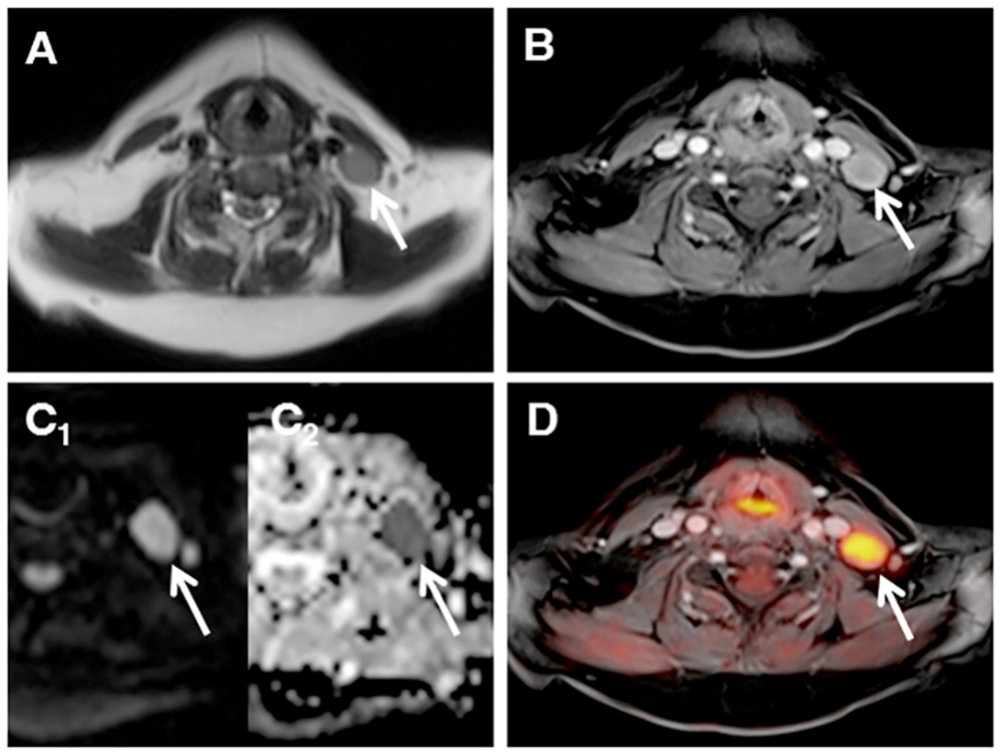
Nodal manifestation of lymphoma (arrows). MR images (T2w Haste, a; T1w VIBE, b) show an enlarged cervical lymph node, which reveals distinctive diffusion restriction (DWI, c_1_; ADC-map, c_2_) as well as pathological glucose metabolism after image fusion in PET/MRI (d).
